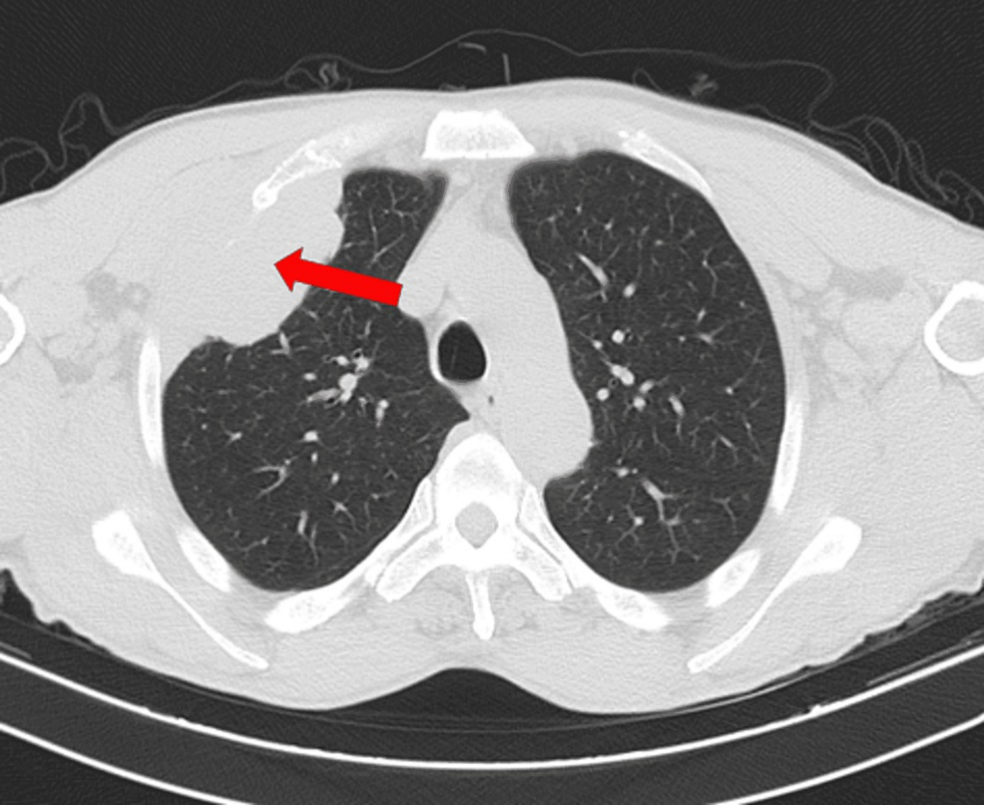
Thoracic CT scan. Right infra-clavicular solid mass, measuring 8 cm, involving and invading the second rib.
